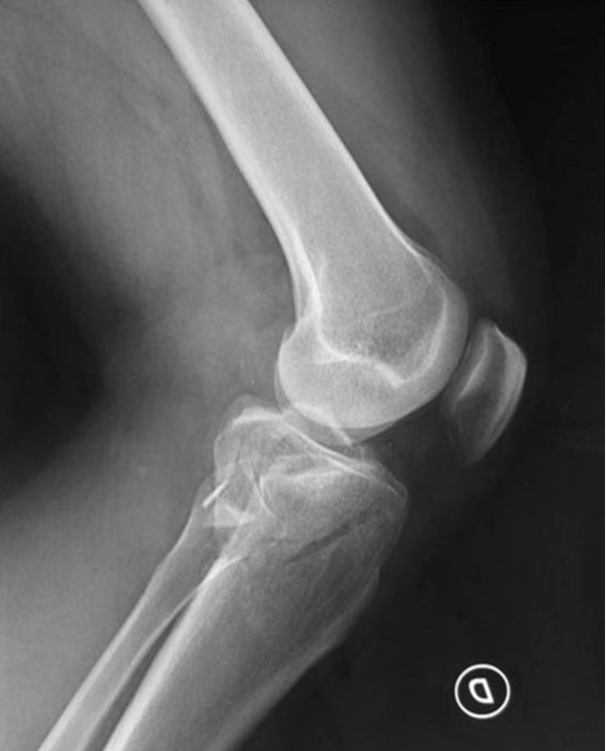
Schatzker type IV fracture, pre-op X-rays (LL)
